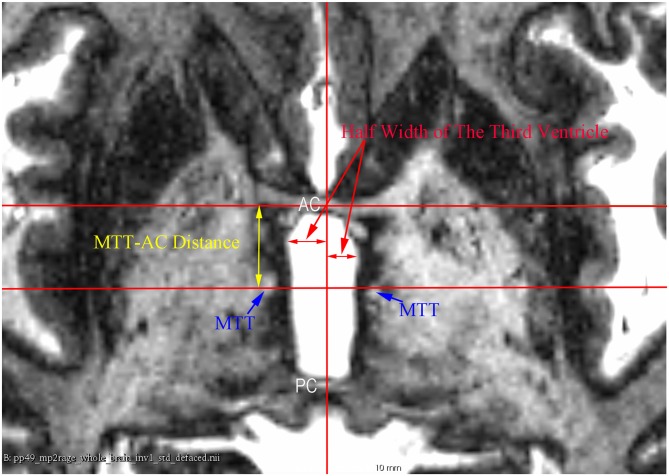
An axial 7 T MR image showing the measurement of the half width of the third ventricle and the mammillothalamic tract (MTT)-AC distance at the AC-PC plane.
